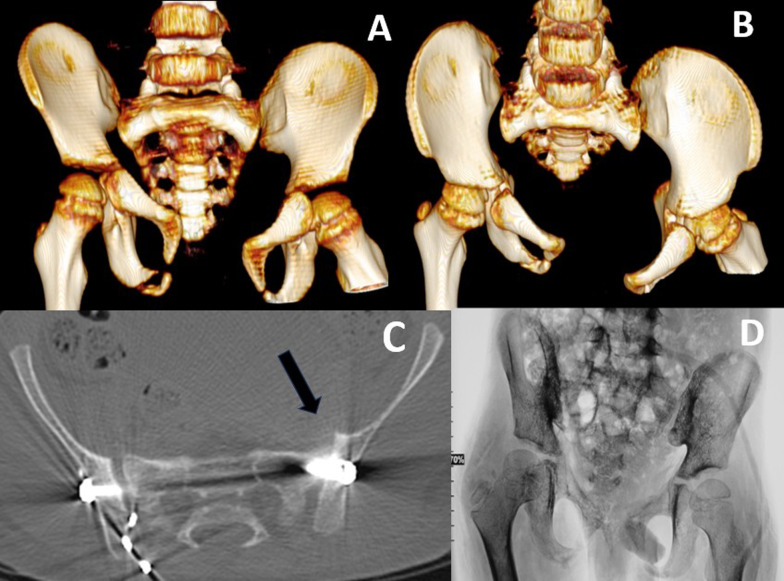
Rolled-over patient with bilateral sacroiliac joint dislocation, more displaced on the right side. It was associated anteriorly with pubic symphysis disruption. A The 3D AP view. B The 3D inlet view. The patient underwent surgical treatment on the right side using an anatomical approach for adequate repositioning of the Risser growth plate. The left side was fixed percutaneously after a closed reduction, without addressing the Risser growth plate. C The imperfect reduction of the sacroiliac joint (*arrow*). D The anteroposterior radiograph at the 5-year follow-up, demonstrating a severe growth disturbance of the iliac wing
